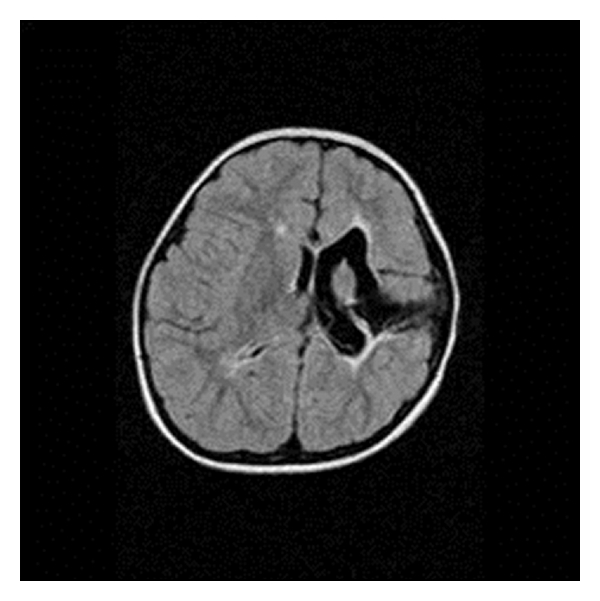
T2W Axial flair MRI image of a 3-year-old boy with infantile hemiplegia seizure syndrome (IHSS).
